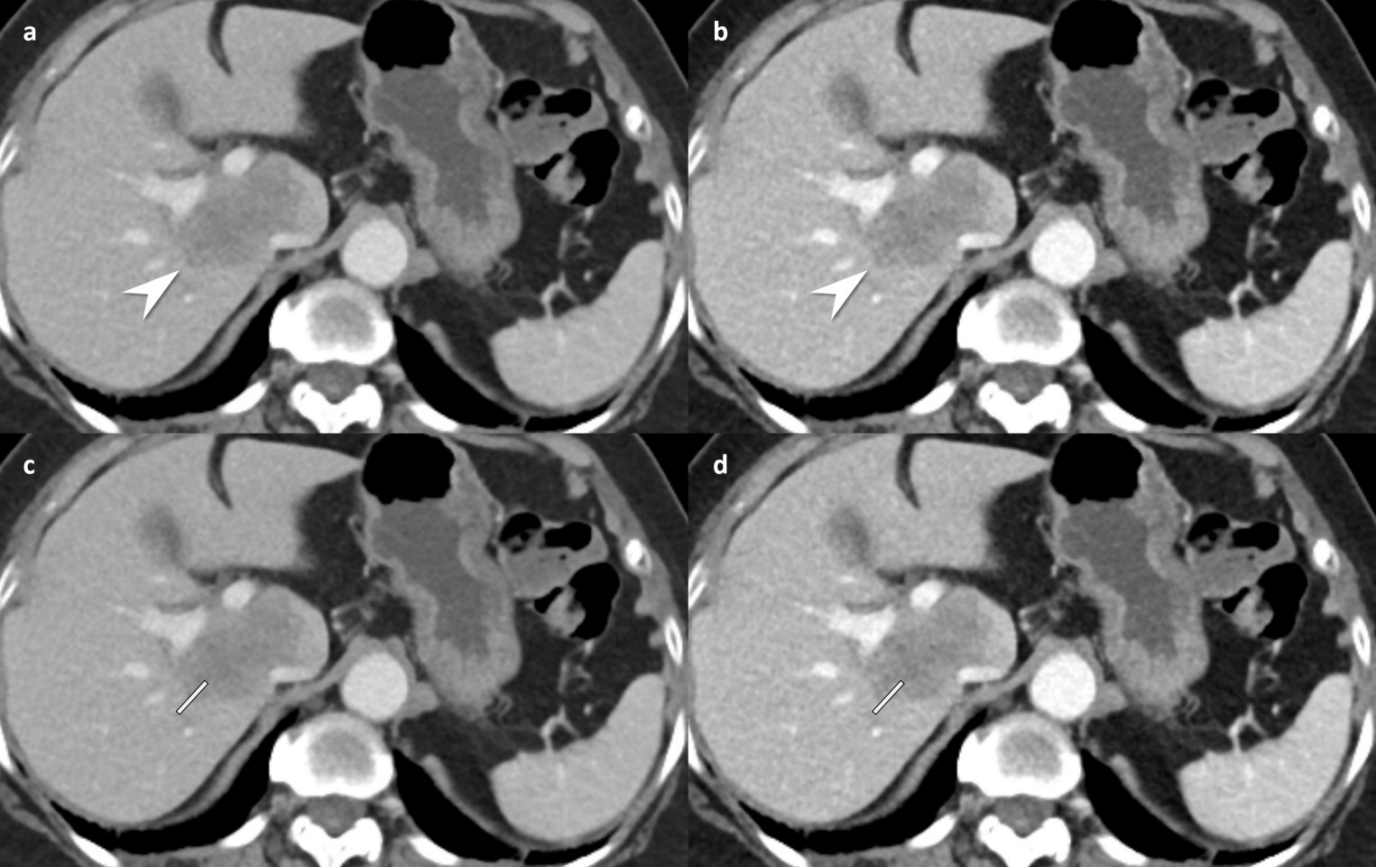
Contrast-enhanced abdomen CT in portal venous phase in a 37-year-old woman (body mass index, 29.0 kg/m^2^) with ascending colon cancer and liver metastasis. 80/Sn150 kVp CT (a, effective dose; 5.9 mSv) shows a liver metastasis in the right hepatic lobe/segment 1 (white arrowhead) by each reader (reader 1 and reader 2, grade 4 lesion conspicuity, grade 5 diagnostic confidence, and grade 5 overall image quality). The corresponding image obtained during the same contrast-enhanced phase with 80 kVp CT (b, effective dose; 3.4 mSv) demonstrates increased conspicuity of the lesion by each reader (white arrowhead, reader 1 and reader 2, grade 5 lesion conspicuity, grade 5 diagnostic confidence, and grade 5 overall image quality). Modified line-density profile revealed higher attenuation differences between the tumor and the normal tissue at 80 kVp (d) than those at 80/Sn150 CT (c) (maximum HU: minimum HU, 157:52 vs.129:49). CT, computed tomography.
